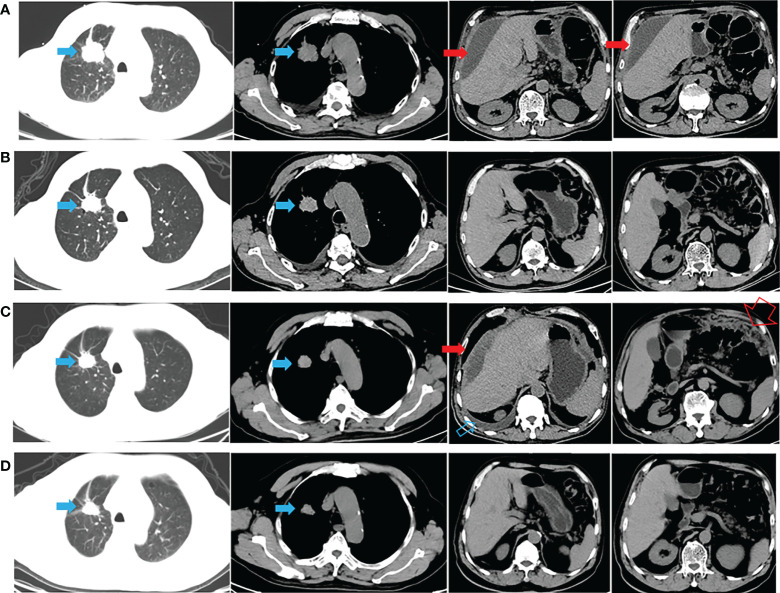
CT appearance across the therapeutic process. (A) Thoracoabdominal CT at diagnosis (after thoracoabdominal drainage); (B) 1 month after almonertinib treatment; (C) hydrothorax and ascites appeared after almonertinib resistance (after thoracoabdominal drainage); (D) hydrothorax and ascites disappeared 1 month after afatinib plus cetuximab treatment. The solid blue arrow indicates the primary lesion; the open blue arrow indicates the hydrothorax; the solid red arrow indicates the malignant ascites; the open red arrow indicates the greater omentum metastasis.
